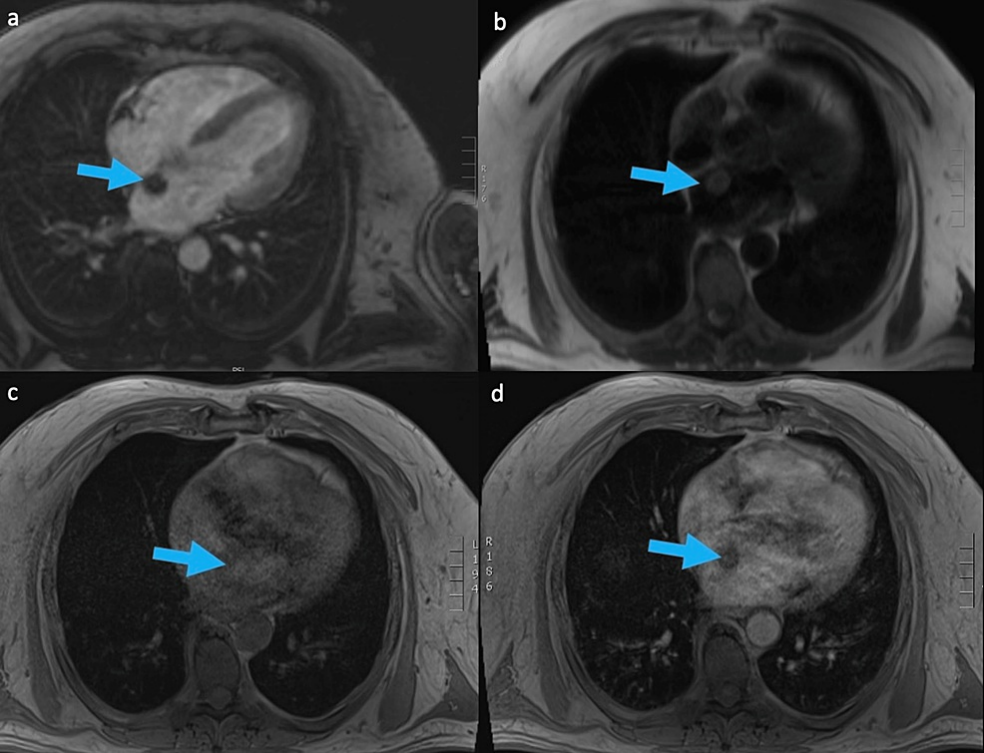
MRI of the heart with and without contrast: (a) four-chamber view, (b) T2 Haste, (c) T1 pre-contrast, and (d) T1 post-contrast (Gd). Pedunculated left atrial mass corresponding with the mass previously seen on CT demonstrating low signal on T1, high signal on T2, and heterogenous enhancement on T1 C+ (Gd) (arrows). MRI: magnetic resonance imaging, CT: computed tomography, Gd: gadolinium
